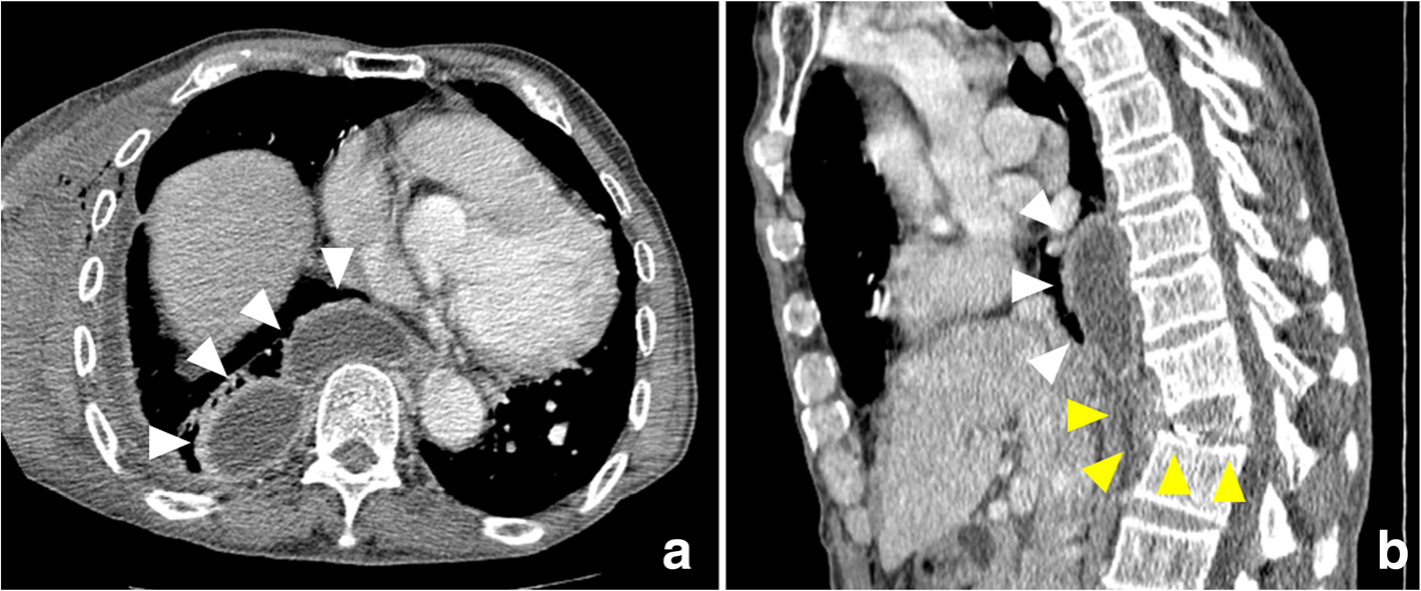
Preoperative enhanced chest computed tomography (CT). Enhanced chest CT revealed a right-sided encapsulated pleural effusion (white arrowheads) and hematoma anterior to the compression fracture of the vertebral body (yellow arrowheads). a Axial view. b Sagittal view
